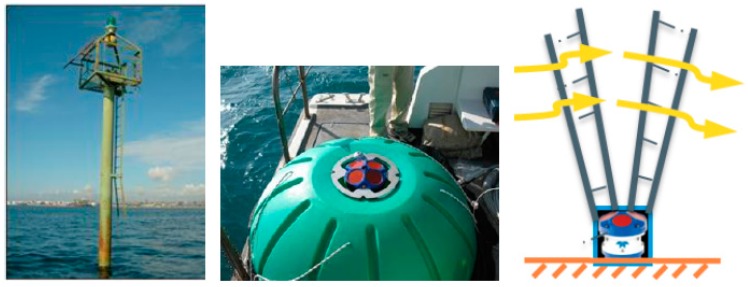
Station in Mar Grande (MG): details of the meteo sensor (left) and of the Acoustic Doppler Current Profiler (ADCP) sensor (central). A sketch of the ADCP functioning is also shown (right).
